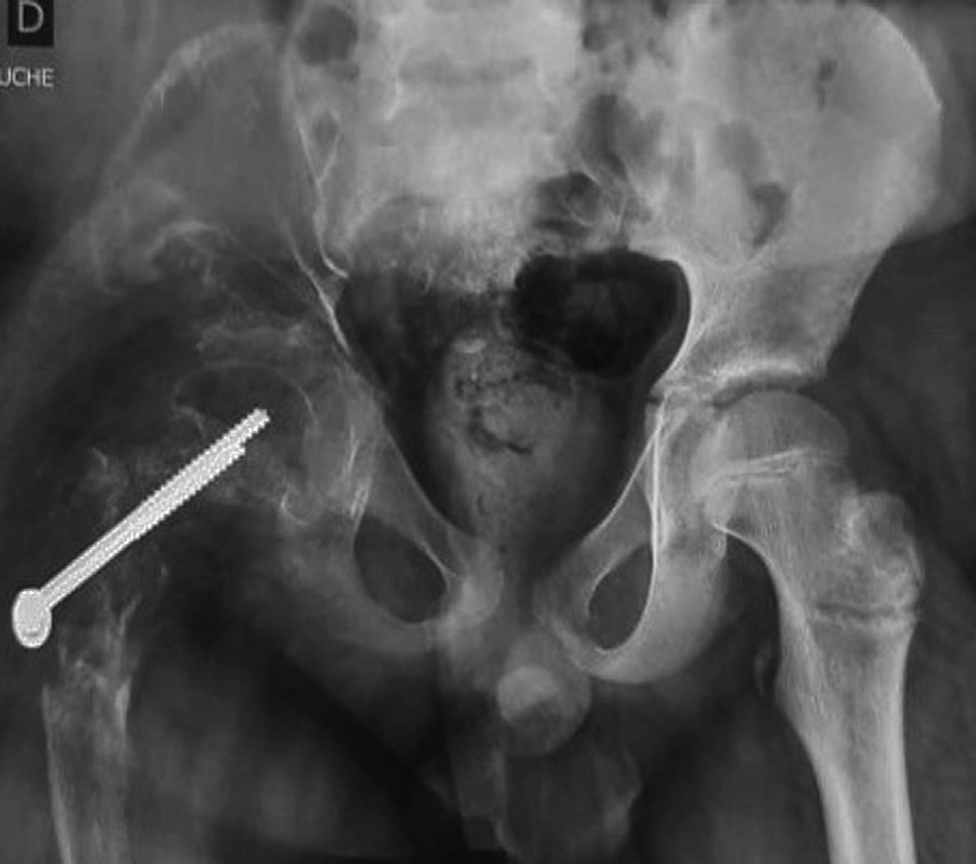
X-ray of the pelvis showing massive osteolysis across the right proximal femur and the iliac crest.
